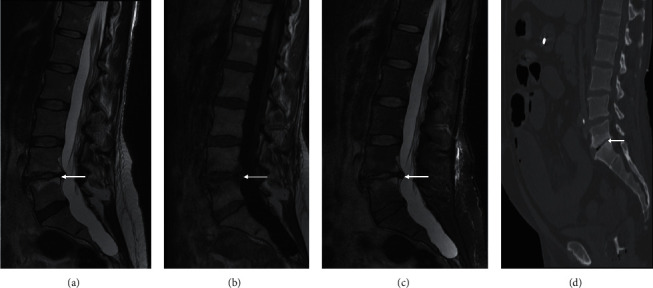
End plate degeneration. Sagittal T2 (a), T1 (b), and STIR (c) lumbar spine of a 40-year-old female presenting with right L5 radiculopathy in the background of low back pain. The fluid-hyperintensity (a, c) and T1 hypointense marrow signal (b) on either side of the L4/5 end plate (arrow), in the context of end plate irregularity and preservation of normal/hypointense disc signal, is consistent with inflammatory or Modic type I degeneration. The radiculopathy was secondary to impingement of the right L5 nerve root from the herniated disc at this level (not shown). Sagittal CT lumbar spine of a different patient (d) shows a vacuum cleft in the L5/S1 disc space with adjacent end plate sclerosis (Modic III degeneration). The vacuum cleft is a useful sign of degeneration.
